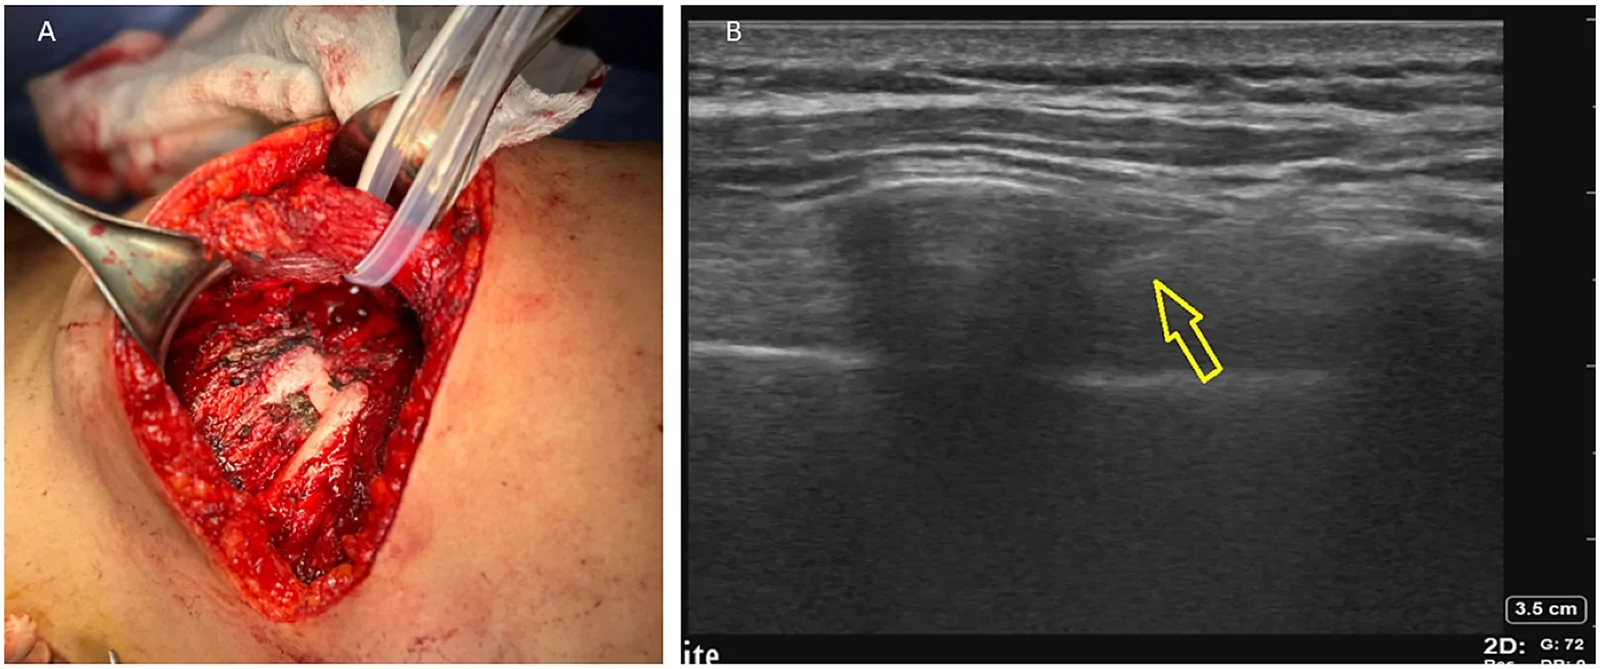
(A) Intraoperative photo showing the heterotopic ossification between ribs 6 and 7; (B) ultrasound-guided RFA with needle tip positioned between the innermost and internal intercostal muscles.
